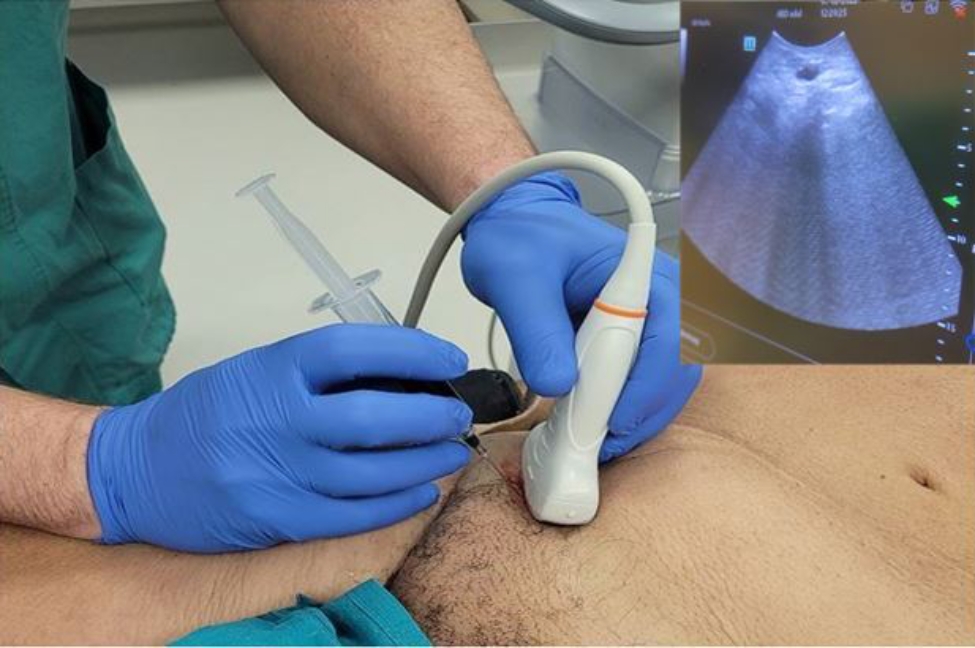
Intra lymph node injection of the ICG solution at the groin under ultrasound guidance
